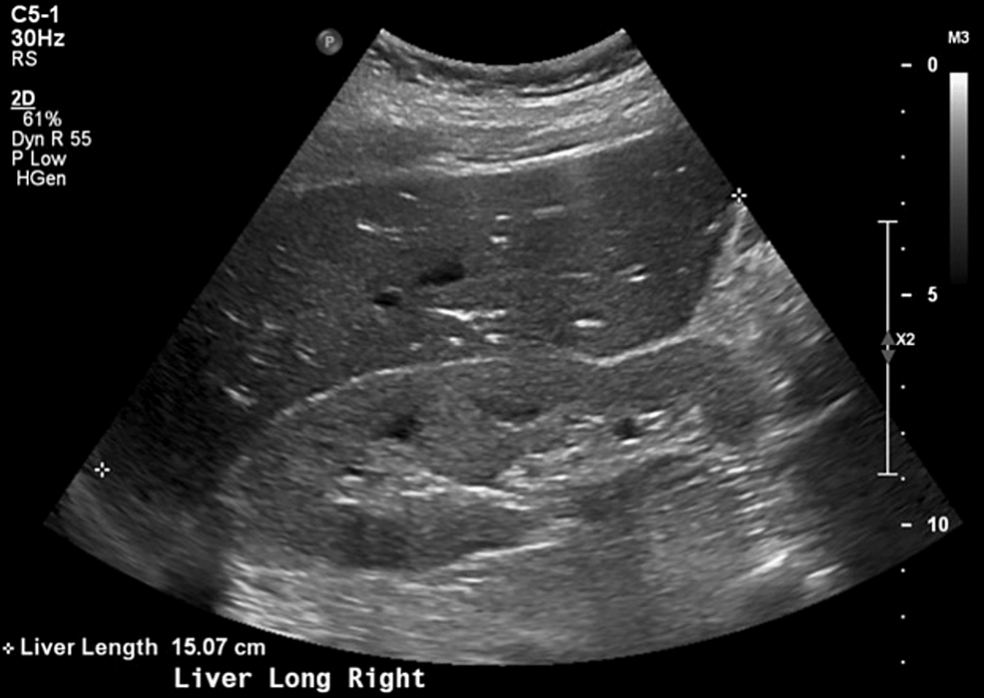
Right upper quadrant ultrasound demonstrating normal echogenicity of the liver with no focal hepatic mass lesions identified
